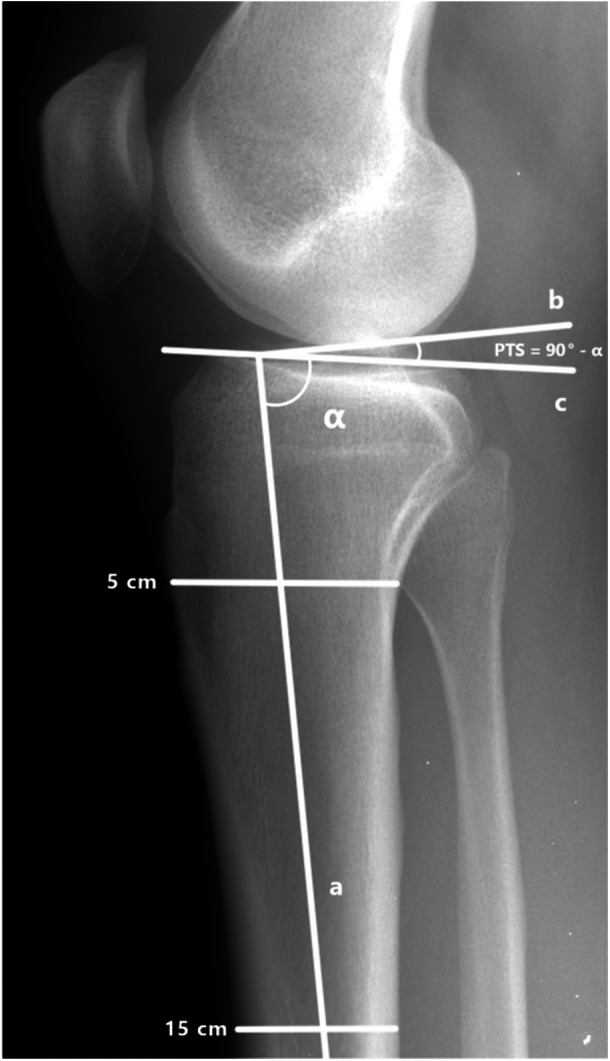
Lateral radiograph of an affected knee at 15°–20° of flexion. Posterior tibial slope (PTS = 90° − *α*) is measured between the perpendicular to the tibial axis (b), defined as the line passing through two points (a), both equidistant from the anterior and posterior tibial cortex (5 and 15 cm distal to the tibial plateau), and the tangent to the anterior and posterior edges of the medial tibial plateau (c).
